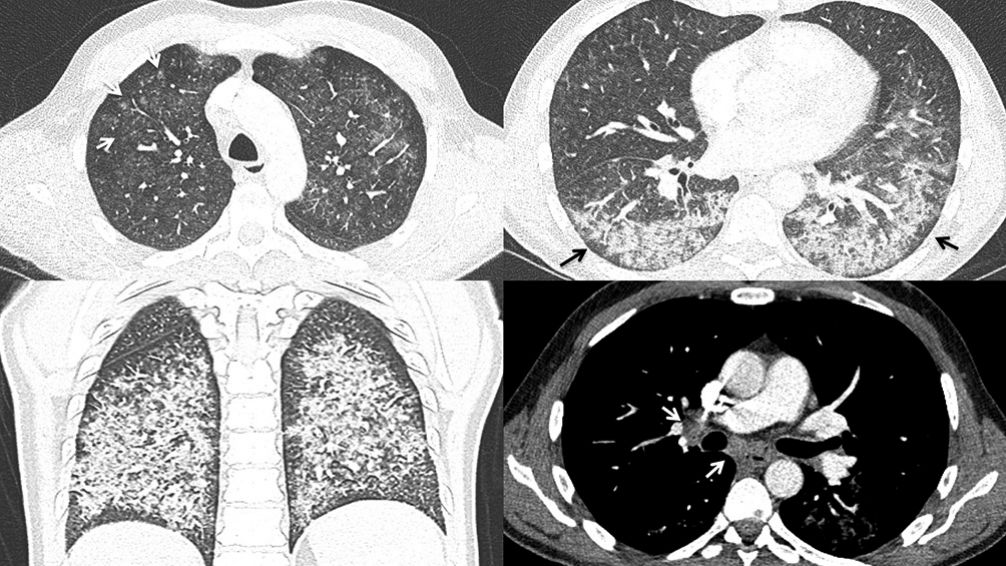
A (top left). Axial HRCT image showing profuse centrilobular groundglass nodules in the upper lobes (white arrows) B (top right). Axial HRCT image showing peribronchovascular airspace opacities in the lower lobes with subpleural sparing (black arrows) C (bottom left). Coronal CT image showing lower lung predominant peribronchovascular airspace opacities D (bottom right). Axial CT image showing mild subcarinal and right hilar lymphadenopathy (white arrows). HRCT, high-resolution CT.
